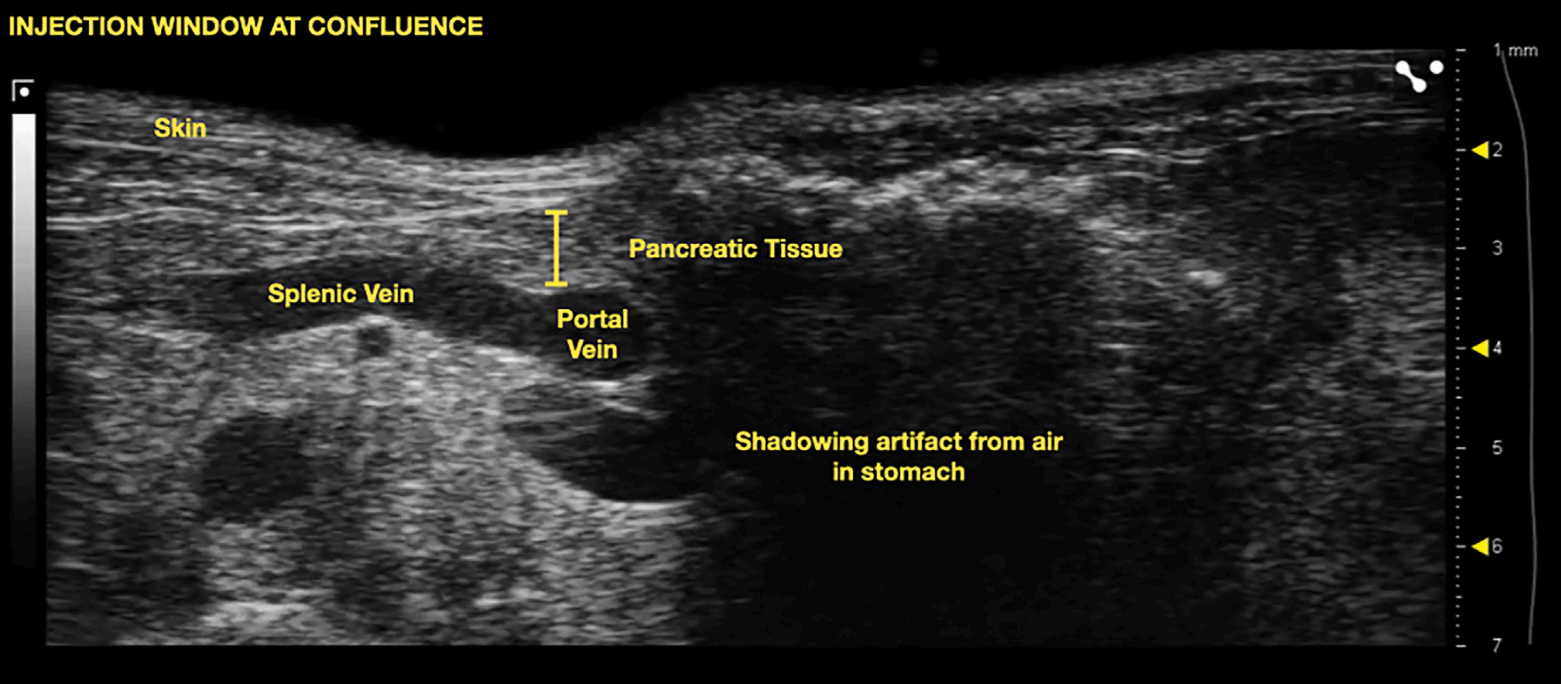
Ideal window configuration at the confluence of the splenic and portal veins*Note:* Adjust the gain by ensuring expected dark regions appear black on the ultrasound screen (blood and fluid should appear darker). Adjust the gain until blood within vessels is black. Similarly, adjust the depth to fill 75% of the screen with the object of interest (the confluence of the splenic and portal veins). This maximizes the quality and crispness of the image.*Optional:* If using the stabilization device, snap the loaded syringe into place and adjust the angle of entry so that the needle tip is positioned over the target. Depending on how thick the gel layer is and how much probe pressure is applied, the probe may need to be raised using the height control to secure the needle to the device. With sufficient space, the needle can be brought into position under the probe. Then, the probe and secured needle can be lowered together into a thick cap of gel. This way, the entire length of the needle can be visualized simultaneously with the target blood vessel. Leaving insufficient space between the probe and the skin risks bending the delicate needle when attempting to position the needle.27.Using freehand technique, position the needle within the gel cap until it is perfectly in plane. An artifact from the needle should be visible (Figures 5 and 6).
